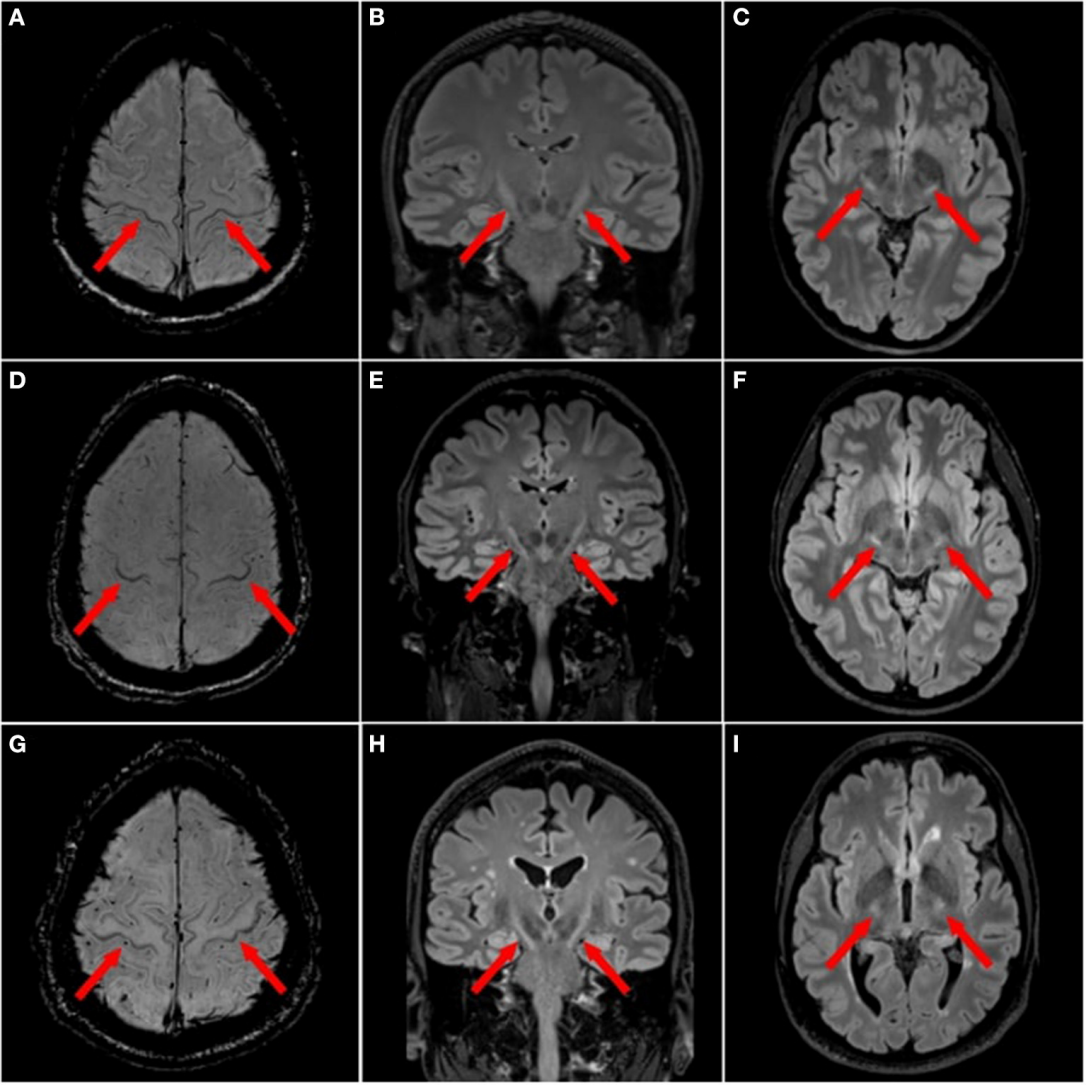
Focal magnetic resonance imaging sign in motor neuron diseases. ALS patients with motor cortex hypointensities, also termed “motor band sign”, on 3T magnetic resonance imaging (MRI) with axial susceptibility weighted imaging (SWI) [(A,D,G) red arrows] and T2 hyperintensities along the corticospinal tract (CST) on 3T 3D T2w-FLAIR in the axial [(B,E,H) red arrows] and coronal planes (C,F,I).
